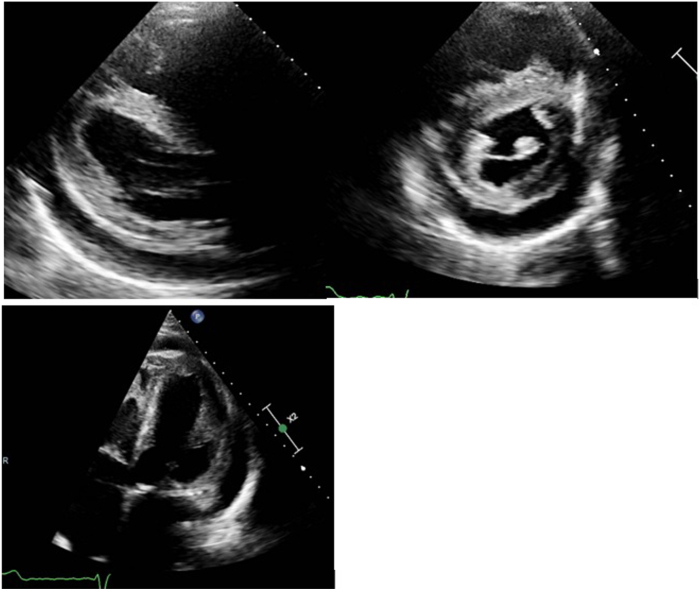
Transthoracic echocardiogram showing left ventricular hypertrophy with global pericardial effusion.
